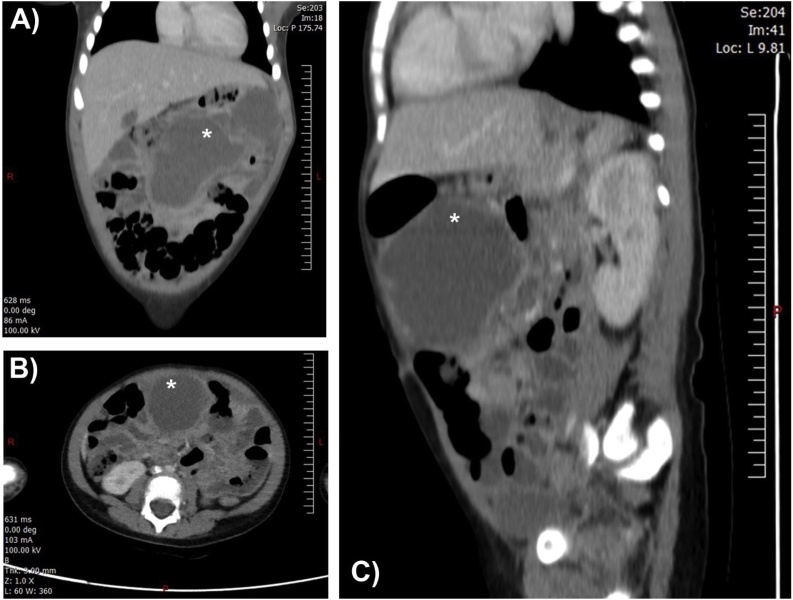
CT scan images at the coronal (A), axial (B), and sagittal planes (C), revealed a multiloculated thick enhancing-walled fluid collection seen in the mesentery anteriorly and extending to the left upper quadrant and flank region.
